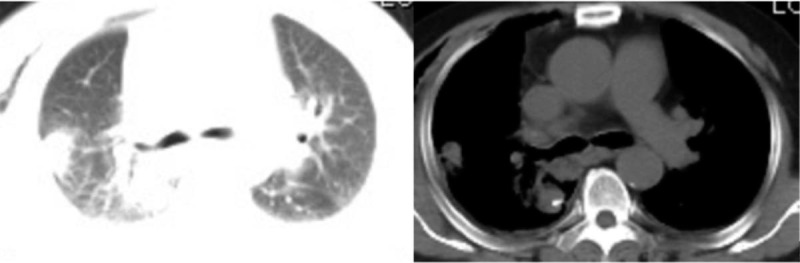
Chest computed tomography (CT) scan the day after surgery: Inflammatory changes in the right lung.
